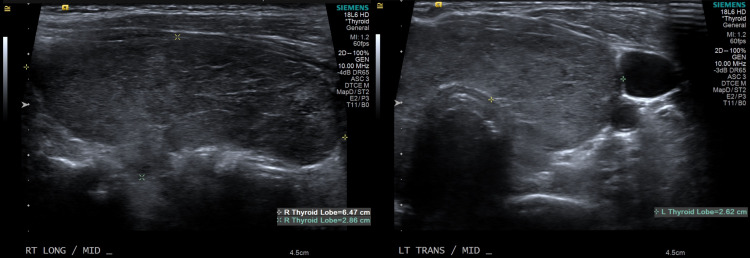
Ultrasound revealed enlarged heterogeneous thyroid gland
